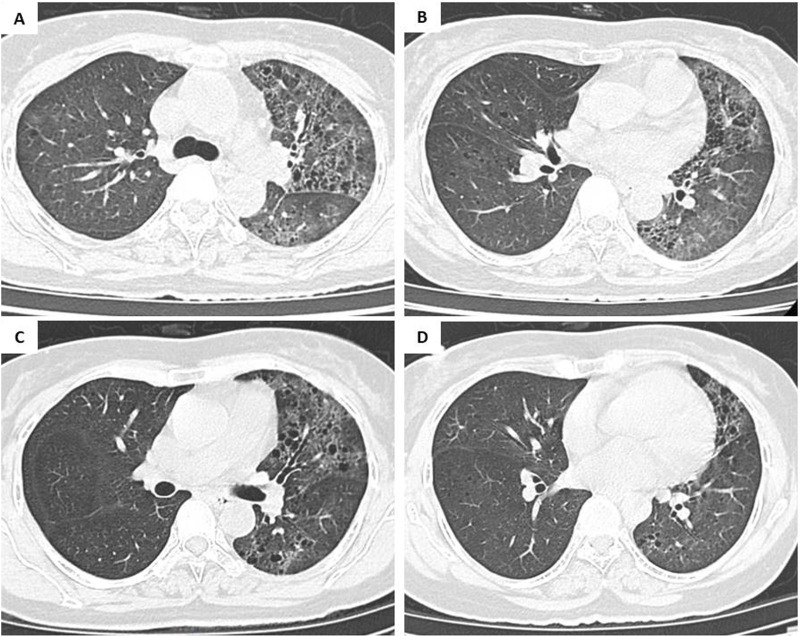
Radiologic response during follow-up. (A,B) Chest CT at 3 months displaying significant reduction in ground-glass opacities and nodular lesions. (C,D) Follow-up scan at 6 months confirming stable disease with no evidence of progression.
